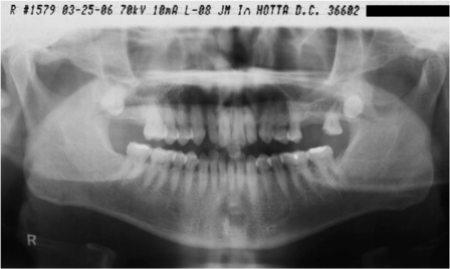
Case 1. March 2006. A panoramic X-ray film obtained before treatment
